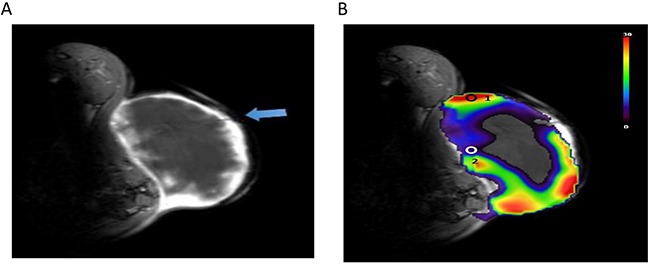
PSIC maps of a representative subcutaneous tumor (A) Gadolinium-enhanced T1-weighted MR image. The blue arrow indicates OxyLite probes. (B) PSIC maps of a representative subcutaneous tumor. Circles represent ROIs; circle “1” indicates the tumor-ROI, and circle “2” indicates the penumbra-ROI.
